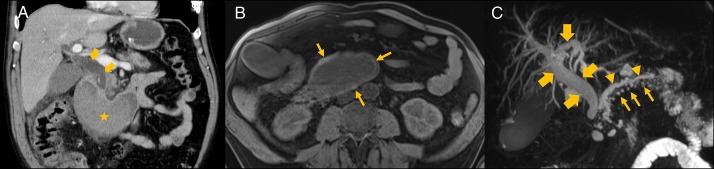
(A) Abdominal computed tomography showing an intramural mass (star) extending from the duodenal knee to the third portion, compressing the duodenal lumen with biliary duct dilatation (arrows). (B) Fat-suppressed T1-weighted axial image reveals textural heterogeneity with hyperintense focuses (arrows) in the periphery of the lesion. (C) Fat-suppressed T2-weighted coronal 3D sequence showing biliary tree dilatation (thick arrows) with irregular dilatation of the main pancreatic duct (arrowheads) and ectatic secondary branches (thin arrows).
